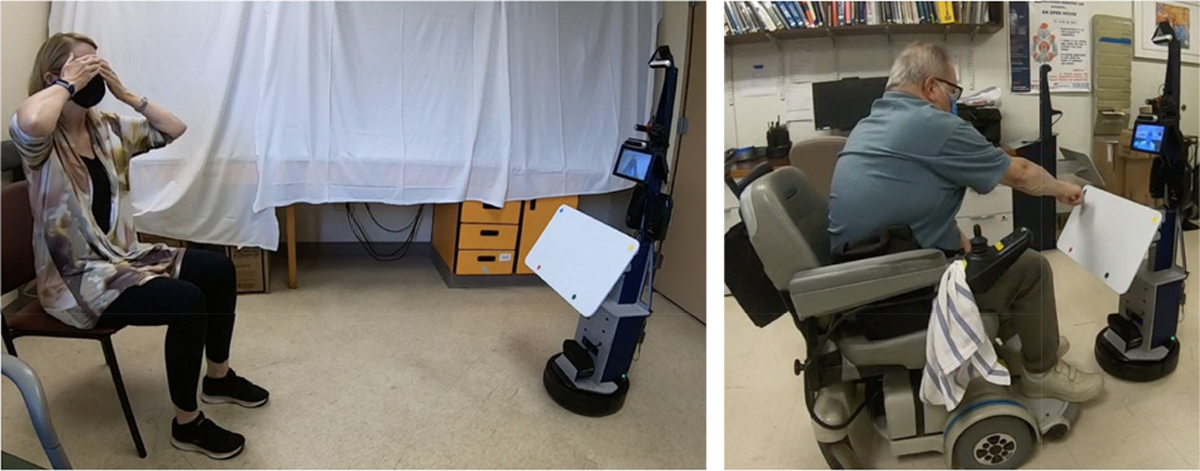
Subjects participating in the classical telepresence (CT) interaction, Simon says on the left and target touch on the right. Subjects shown provided release to publish images of them.
